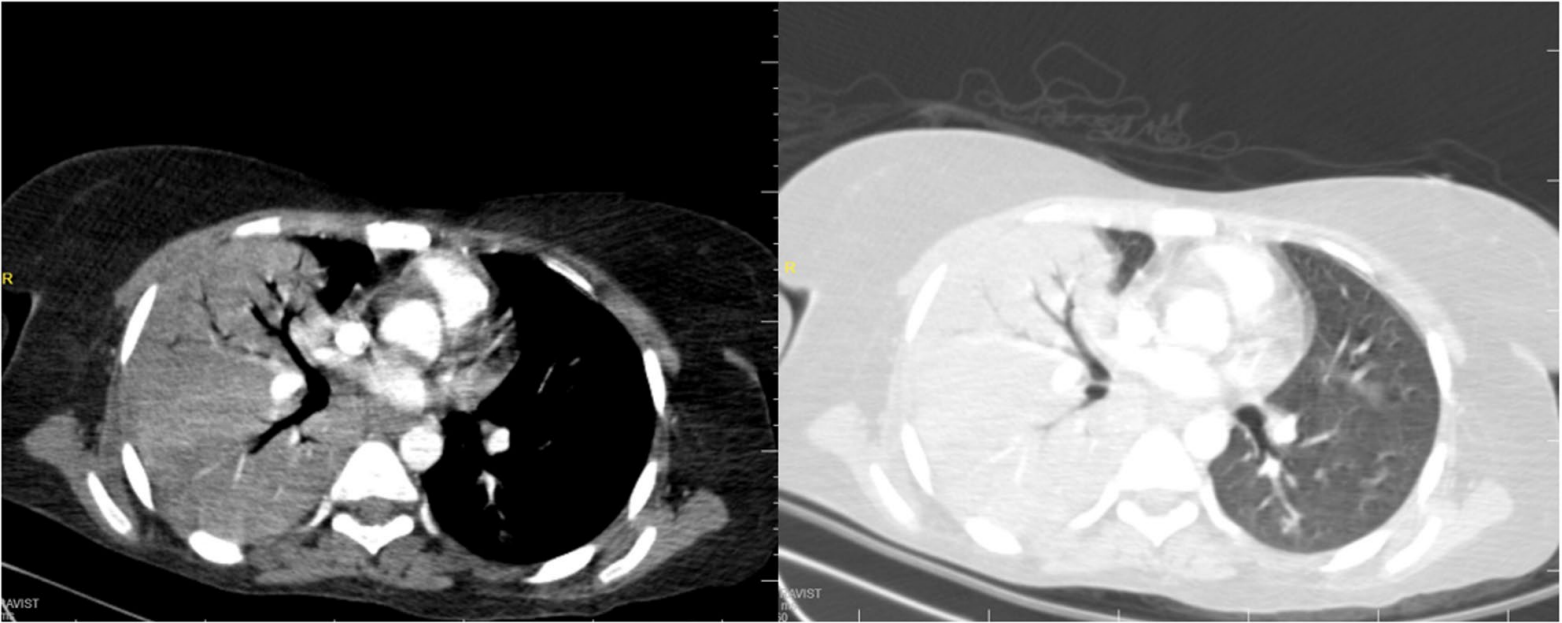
Chest computed tomography demonstrating extensive aspiration pneumonia, characterized by confluent consolidation with prominent air bronchograms involving the majority of the right lung, associated with a small right-sided pleural effusion. Additional discrete areas of consolidation are noted within the left upper and lower lobes
